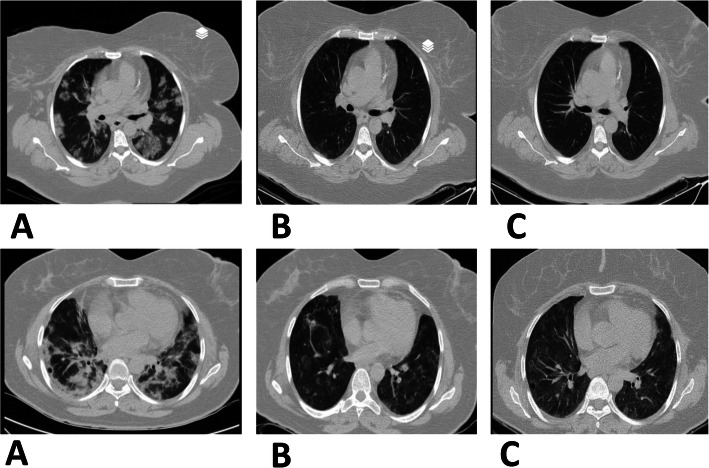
Lung CT scan. a (A–C) There are multifocal patchy alveolar opacities in both lungs that in follow-up exam 14 days and 30 days later disappeared following stem cell therapy. P1: Patient 1. b (A–C) There are multifocal patchy alveolar opacities in both lungs that in follow-up exam 14 days and 30 days later disappeared following stem cell therapy. P2: Patient 2
